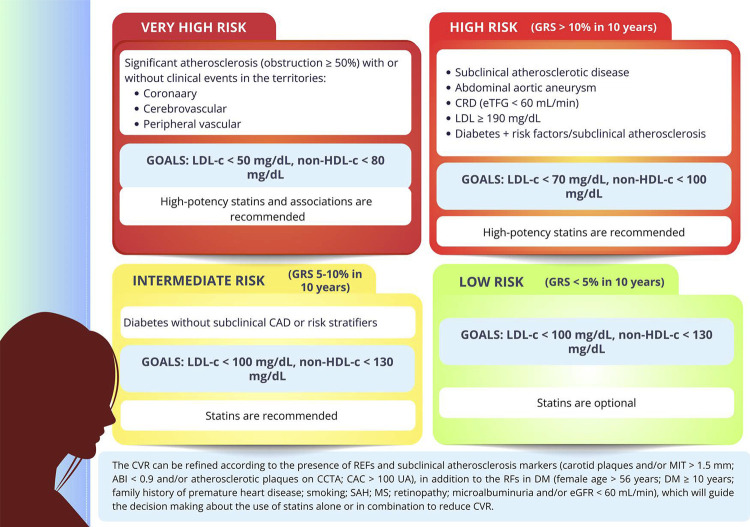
Cardiovascular risk stratification and therapeutic goals for postmenopausal and menopausal women. Adapted from Oliveira et al.13 ABI: ankle-brachial index; CAC: coronary artery calcium score; CAD: coronary artery disease; CCTA: coronary computed tomography angiography; CRD: chronic renal disease; CVR: cardiovascular risk; DM: diabetes mellitus; eGFR: estimated glomerular filtration rate; GRS: global risk score; MIT: medio-intimal thickening; MS: metabolic syndrome; RF: risk factor; REF: risk-enhancing factors; SAH: systemic arterial hypertension.
